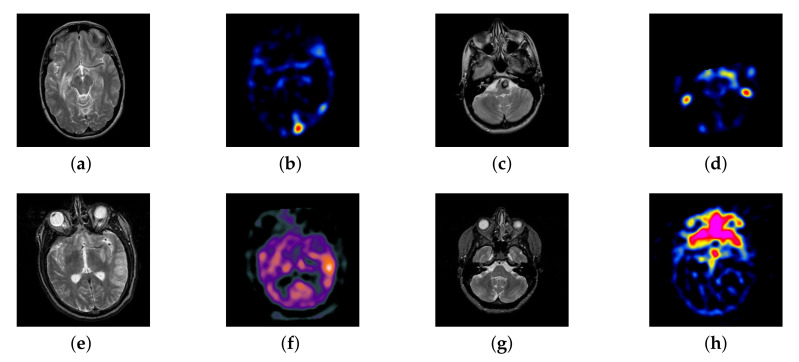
Four pairs of magnetic resonance imaging (MRI)-single-photon emission computed tomography (SPECT) source images: (a,c,e,g) are MRI images; (b,d,f,h) are SPECT images.
